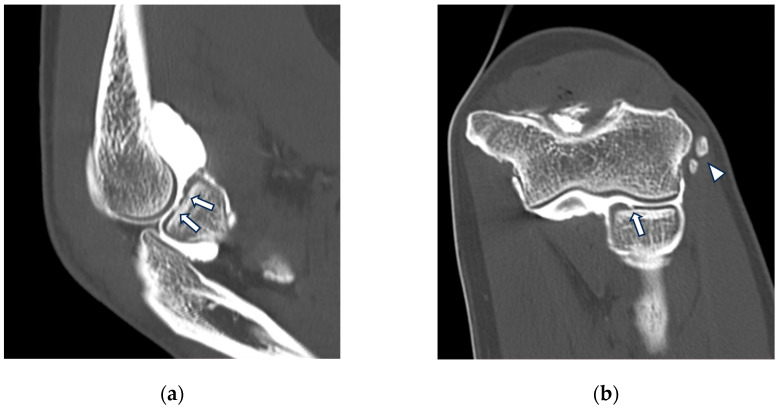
CT arthrography of a patient with chronic elbow instability. (a) Sagittal image shows pitting and fissuring of radial head cartilage, involving >50% of its thickness (grade III) (white arrows); (b) coronal reformat of the same patient shows a focal full-thickness cartilage defect of the anteromedial radial head (grade IV) (white arrow). In the same image, mature calcifications can be seen at the insertion of the common extensor tendon (white arrowhead).
